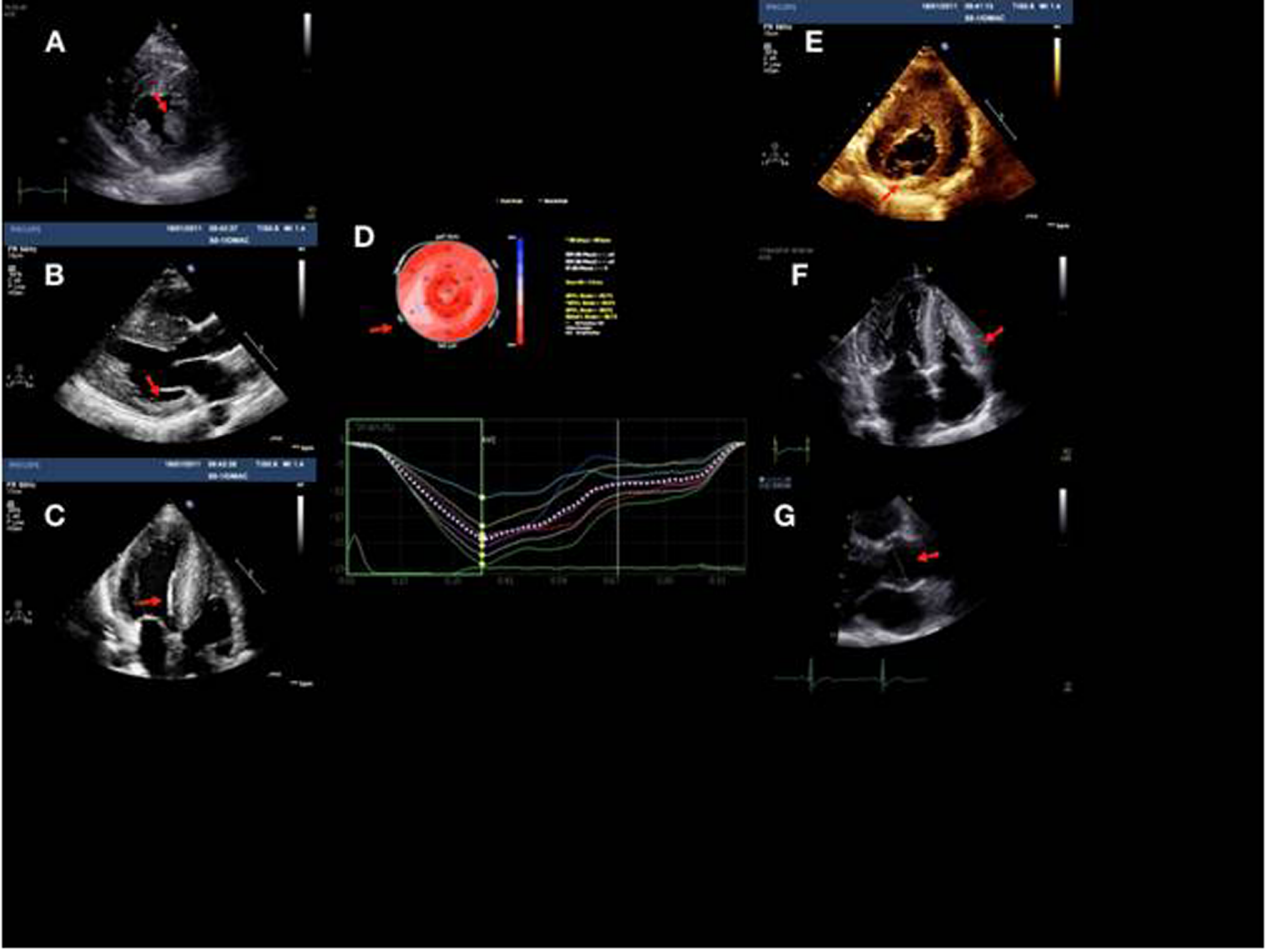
Echocardiography findings in a patient with Fabry disease. A Hypertrophic papillary muscle, B thinning in inferior and posterior basal walls of the left ventricle in an advanced case, C “Binary sign”, D typical “Strain Bull’s Eye” in FD, segmenter longitunal strain reduction in infero-basal wall, E increase echogenicity in areas with advanced myocardial fibrosis, F right ventricular hypertrophy and valvular thickening, G Aortic dilation at Valsalva sinuses
